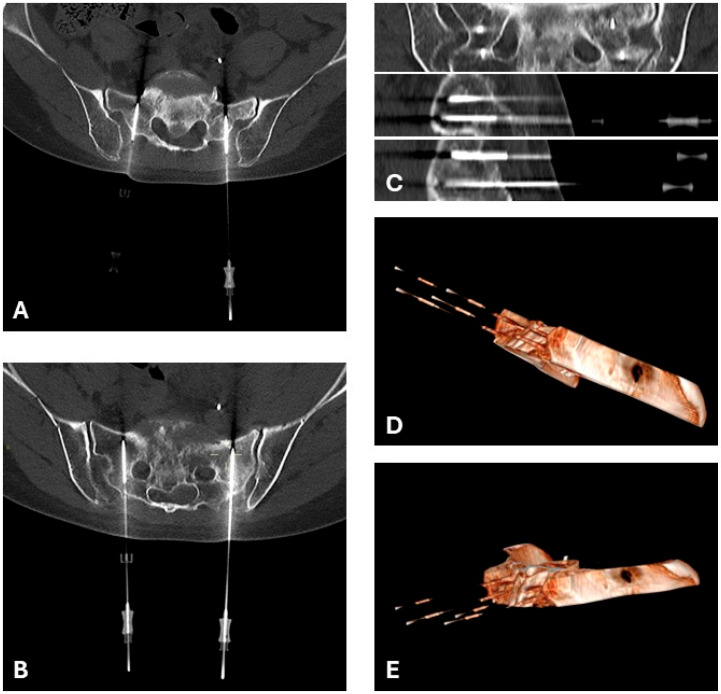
A 51-year-old female, affected by colon adenocarcinoma, presented at our Institute with an osteolytic lesion of the sacrum. The patient was treated with electrochemotherapy. CT scans (A,B) and 3D (D,E) reconstructions show the percutaneous positioning of the four 18 VGD needle electrodes (30 mm active tip) following a parallel geometry (C), allowing the entire lesion to be treated. Bleomycin was injected intravenously, and electroporation was performed. No adverse events were recorded after the procedure. The patient reported clinical improvement at the 3-month follow-up.
